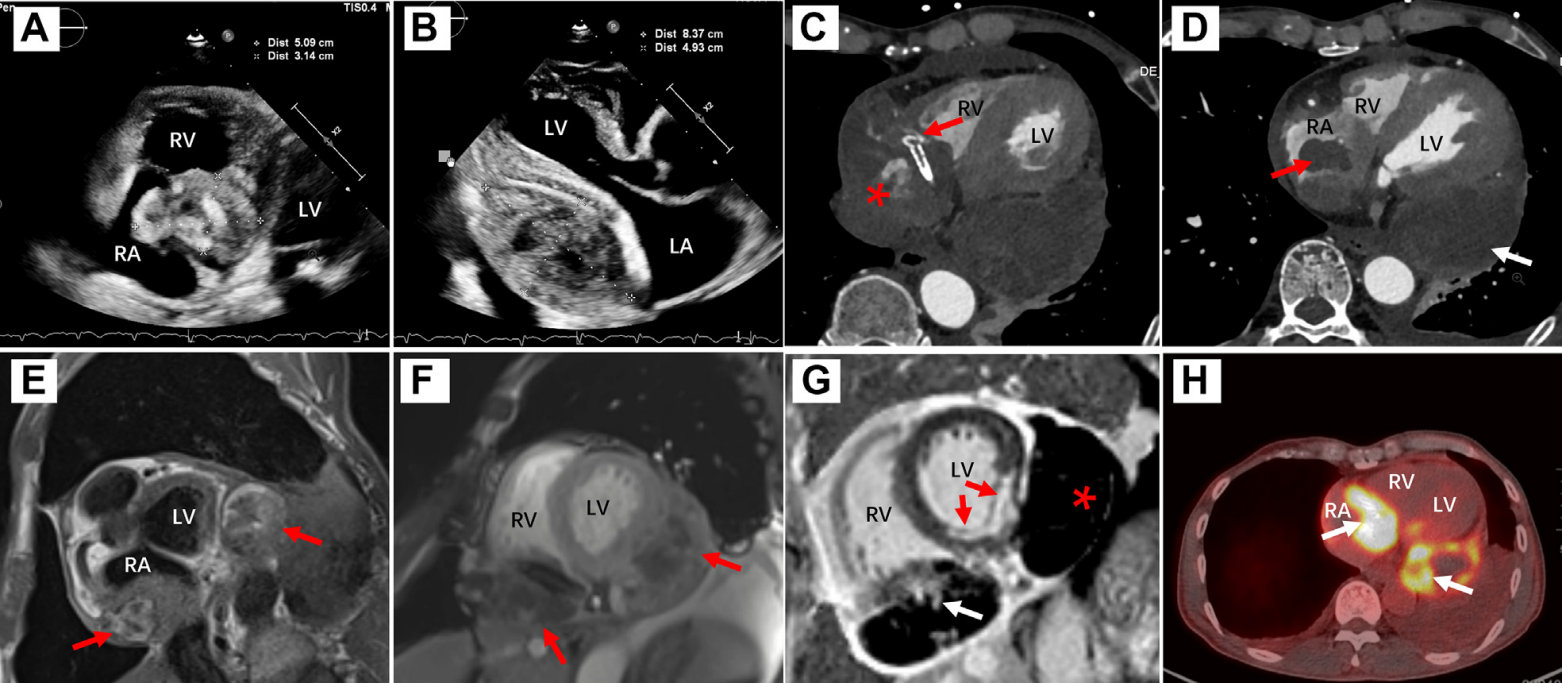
Multimodality Imaging of Cardiac Angiosarcoma TTE revealed a heterogeneous 5.09- × 3.14-cm mass located on the lateral wall of the RA (A) and an 8.37- × 4.93-cm hypoechoic mass on the lateral wall of the LV (B). CT showed the heterogeneous mass in the lateral wall of the RA encased the RCA, with stent angulation (C, red arrow) and contrast extravasation (C, asterisk), with partial invasion into the right atrial cavity (D, red arrow). The well-defined mass on the posterior wall of the LV had extensive pericardial adhesions, but without contrast enhancement (D, white arrow). T1-weighted imaging (E) and cine imaging (E) in CMR demonstrated that mixed T1 signal was noted in both masses (red arrows). LGEs were detected in the mass on the lateral wall of the RA (G, white arrow), but not in the mass on the posterior wall of the LV (G, asterisk). Transmural LGE was noted in the inferior septal and inferior wall (G, red arrows). PET-CT indicated increased FDG metabolism in both masses (H, white arrows). CT = computed tomography; CMR = cardiac magnetic resonance; FDG = fluorodeoxyglucose; LA = left atrium; LGE = late-gadolinium enhancement; LV = left ventricle; PET-CT = positron emission tomography–computed tomography; RA = right atrium; RCA = right coronary artery; RV = right ventricle; TTE = transthoracic echocardiography.
